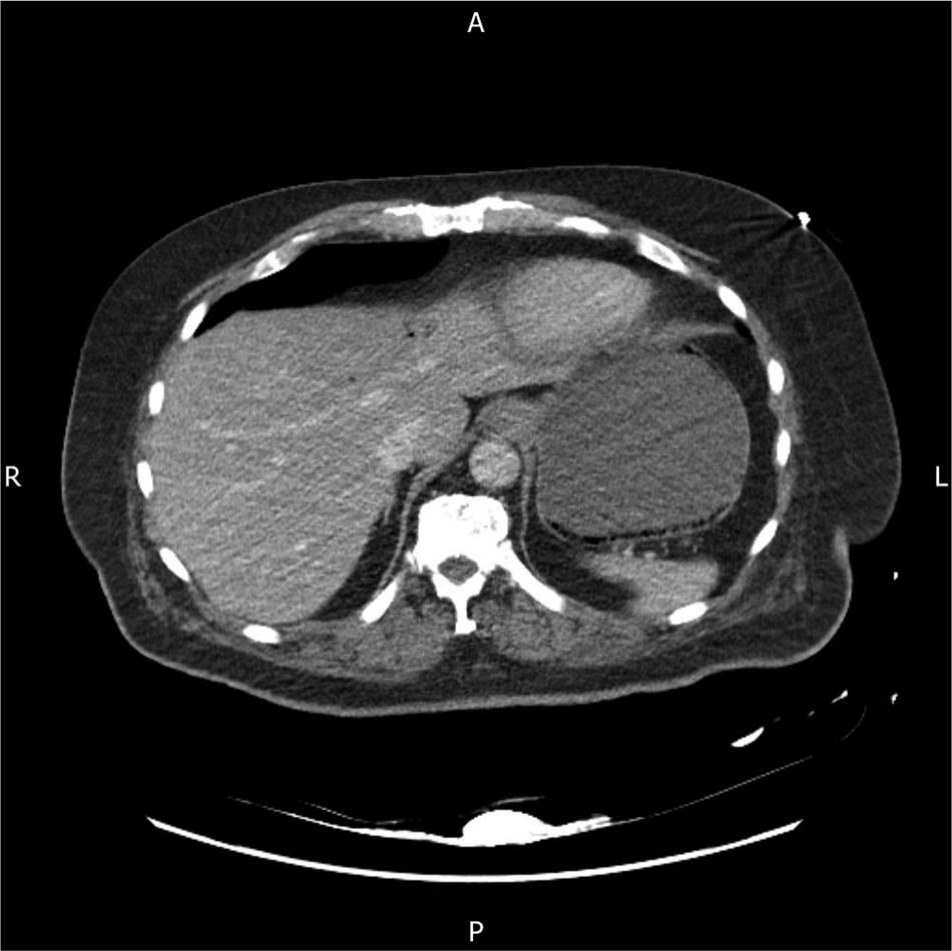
Axial contrast-enhanced CT abdomen on admission showing mottled intramural gas within the gastric wall and associated hepatic portal venous gas in the left lobe, in keeping with emphysematous gastritis.
